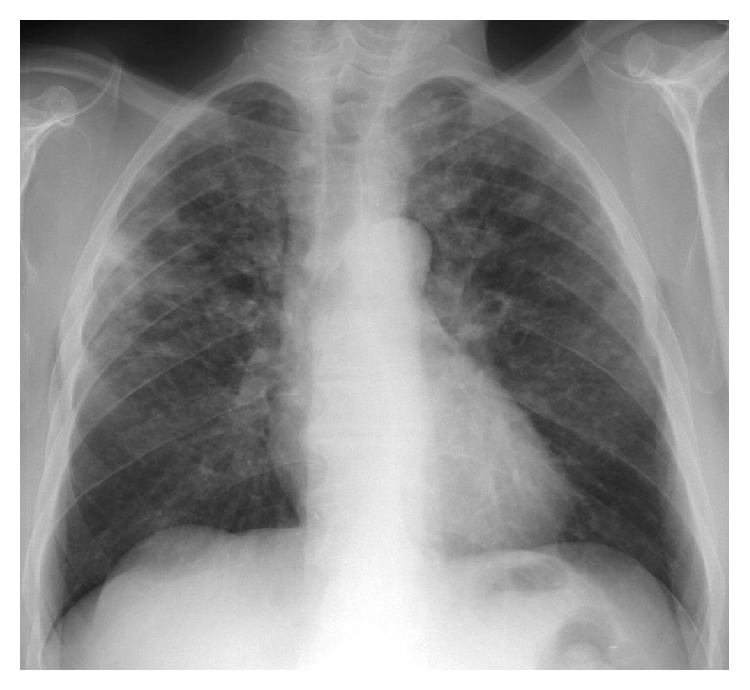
Thoracic X-ray: multiple pulmonary nodules are identified, with upper lobe predominance. Note that some nodules show cavitation.
